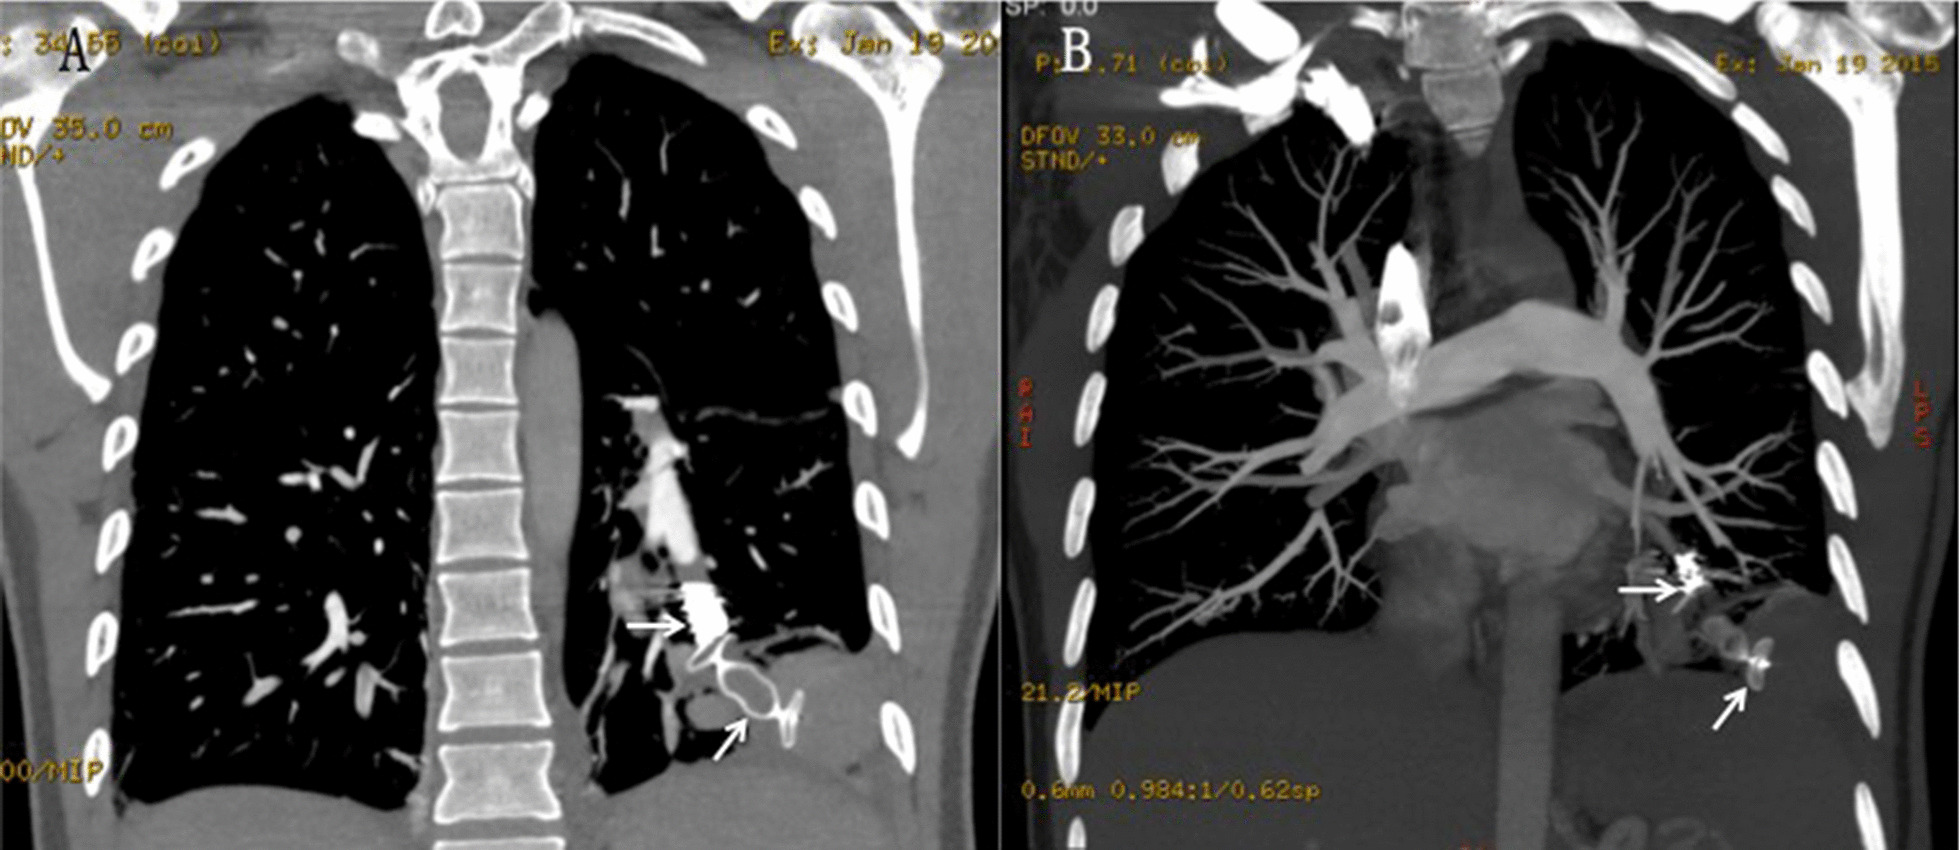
Images from the 6-month follow-up CTA and 3-dimensional reconstruction show that the sac disappeared completely, and the Amplatzer vascular plug and coil (arrow) showed a typical appearance
